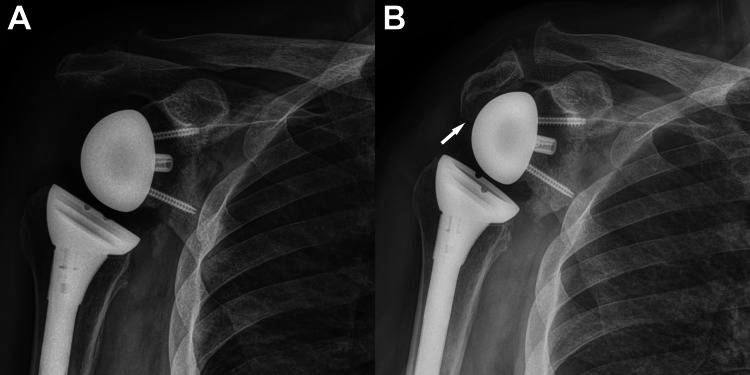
Anterior-posterior radiographs of right RTSA. A. Our patient’s RTSA at two months (March 2020) after implantation surgery (before the acromion fracture). B. His RTSA showing the acromion fracture (November 2021); note the narrowed subacromial space (arrow). RTSA, reverse total shoulder arthroplasty
